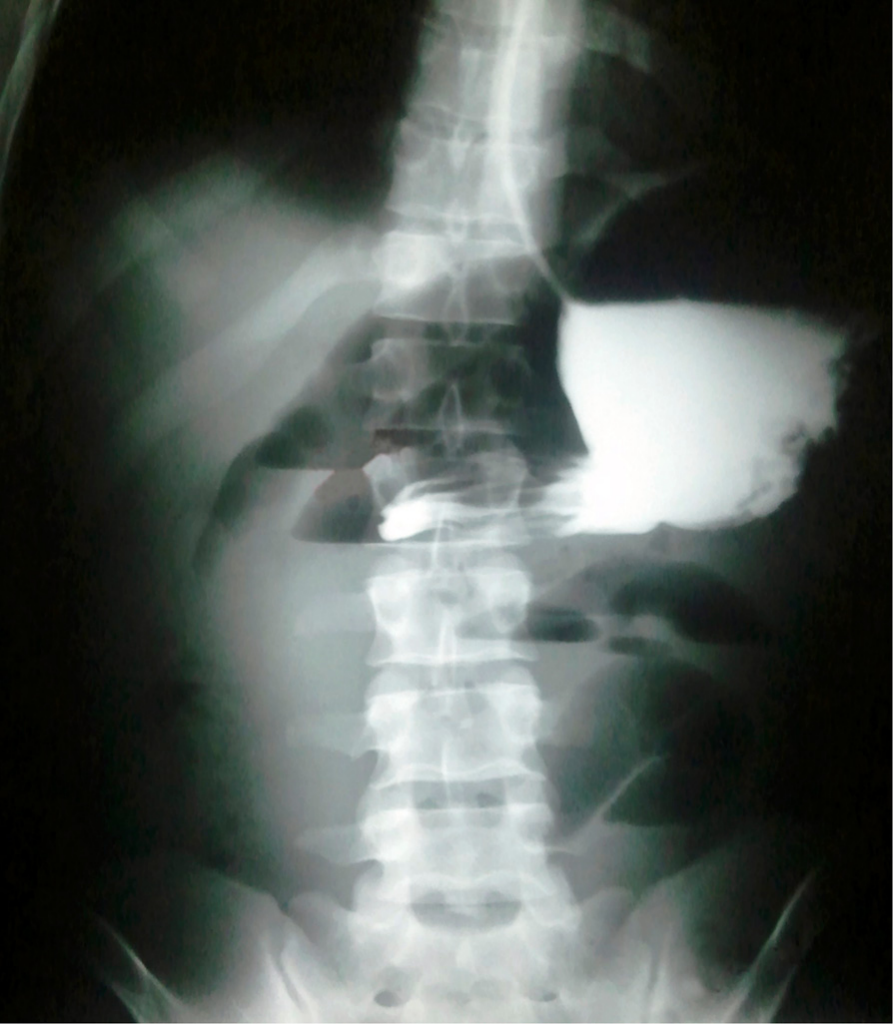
Abdominal X-ray after Gastrografin (sodium diatrizoate and meglumine diatrizoate) administration suggesting obstruction of upper gastrointestinal tract.
